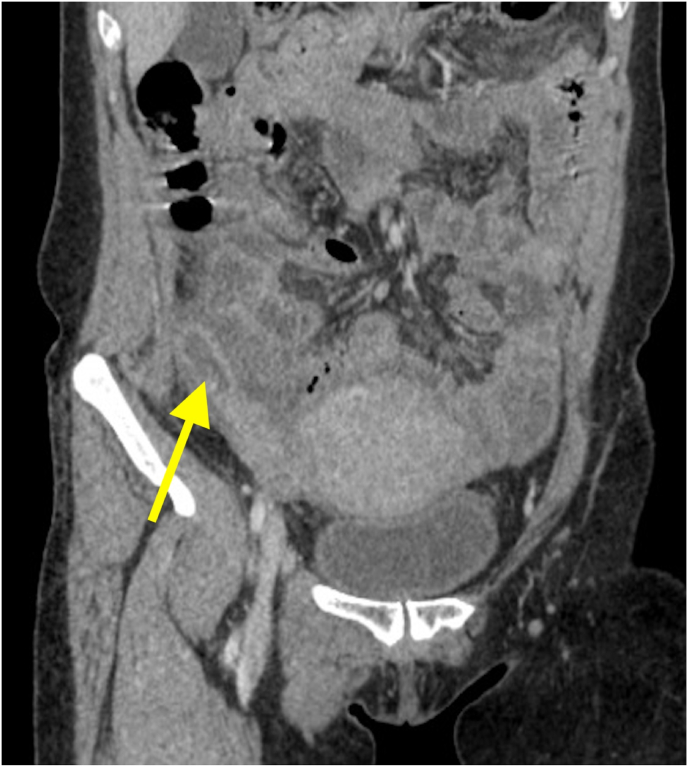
A coronal non-contrast CT scan.
